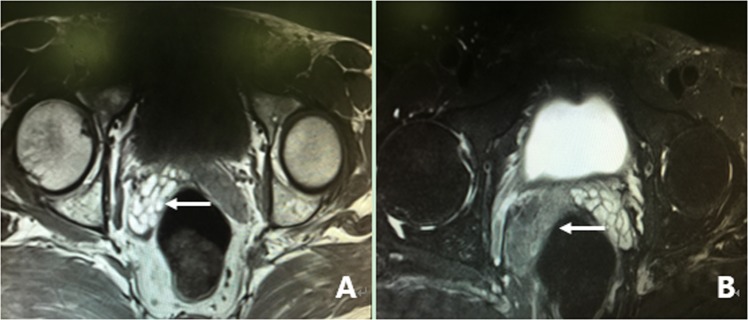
MRI image of a fresh hemorrhage in the right side of the SV. (A) This image shows high signal intensity in the right SV and low signal intensity in the left SV on T1WI. (B) This image shows low signal intensity in the right SV and high signal intensity in the left SV on T2WI. This pattern suggests that a fresh hemorrhage is present in the right SV.
